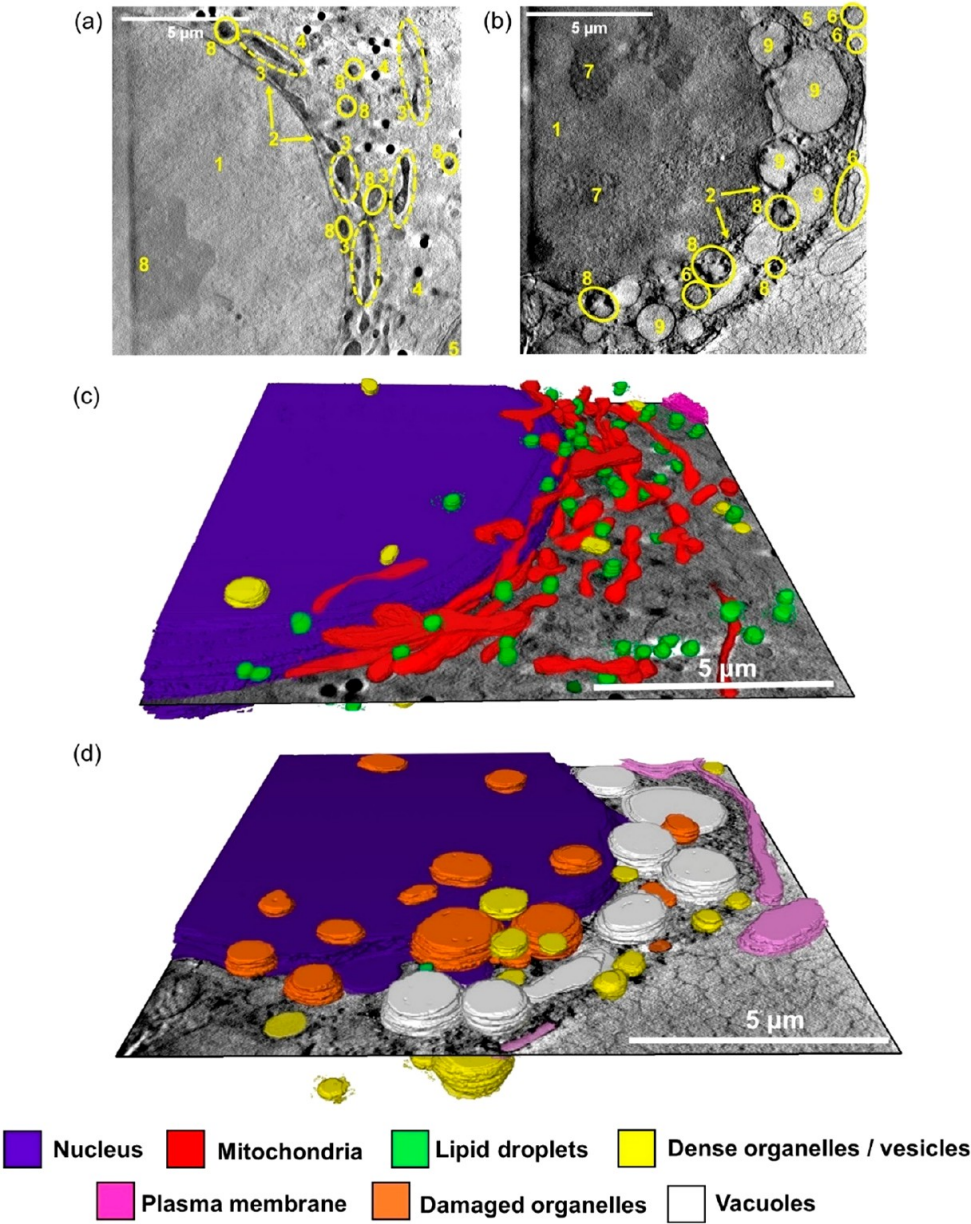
Reconstructed X-ray tomograms and 3D segmented tomograms of two
